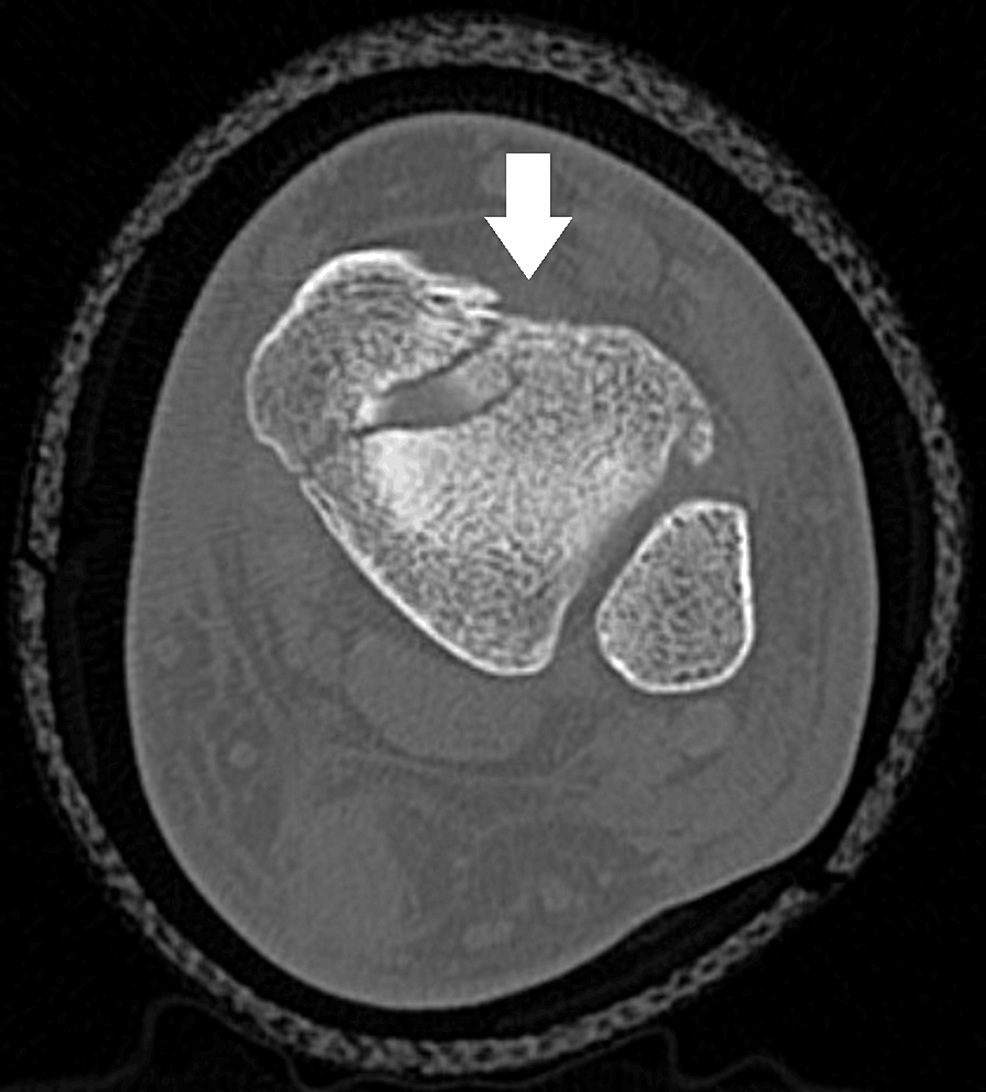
Axial view on CT scan of the medial malleolar fracture with an arrow indicating the location of the fracture. CT: computed tomography
